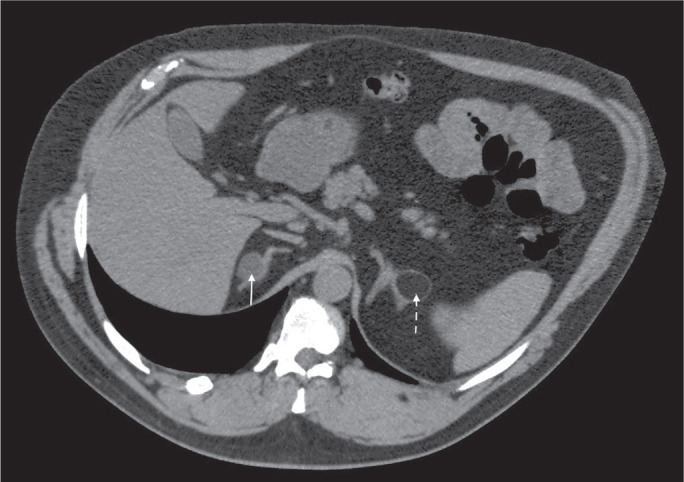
A 54-year-old male patient with Crohn’s disease. Axial oblique CT
